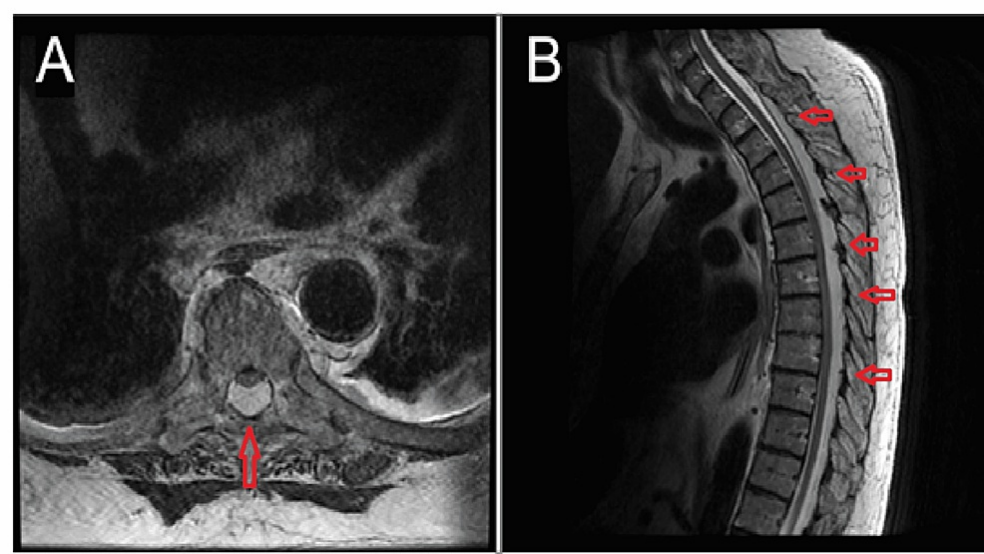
Pre-operative T2-weighted MRI revealing significant epidural lipomatosis with anterior thecal sac displacement and cord compression. A) axial view, B) sagittal view
